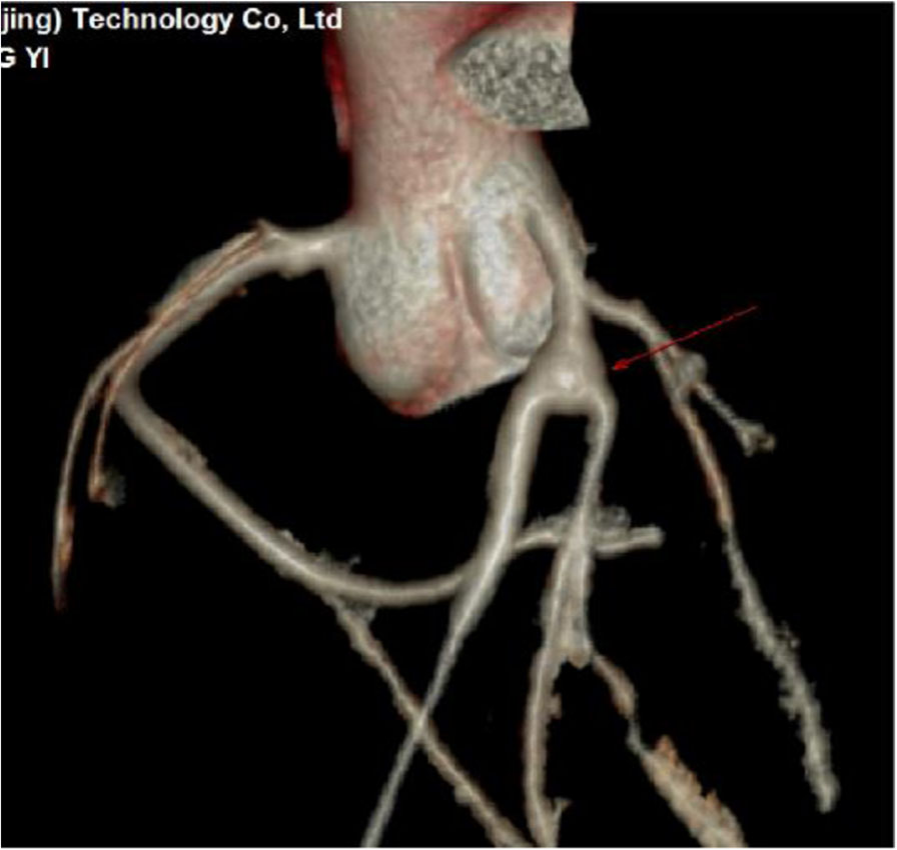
CTA showing a very large round aneurysm originating from the proximal segment of the LAD (red arrow indicating the aneurysm)
